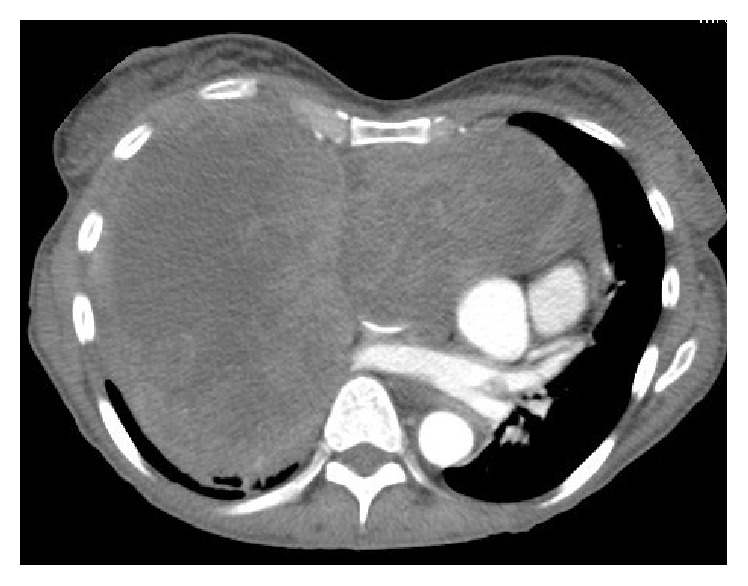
Axial CT at 3 months demonstrating progressive disease.
